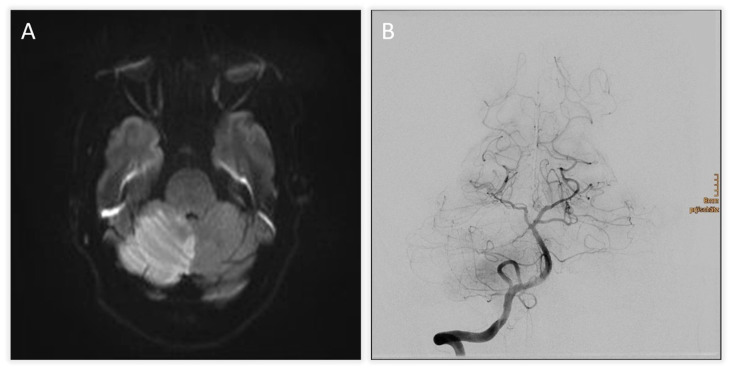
(A) Diffusion-weighted MRI on admission showing acute ischemic infarction of the right cerebellar hemisphere and vermis. (B) DSA demonstrating occlusion of the right SCA with preserved patency of the basilar tip.
